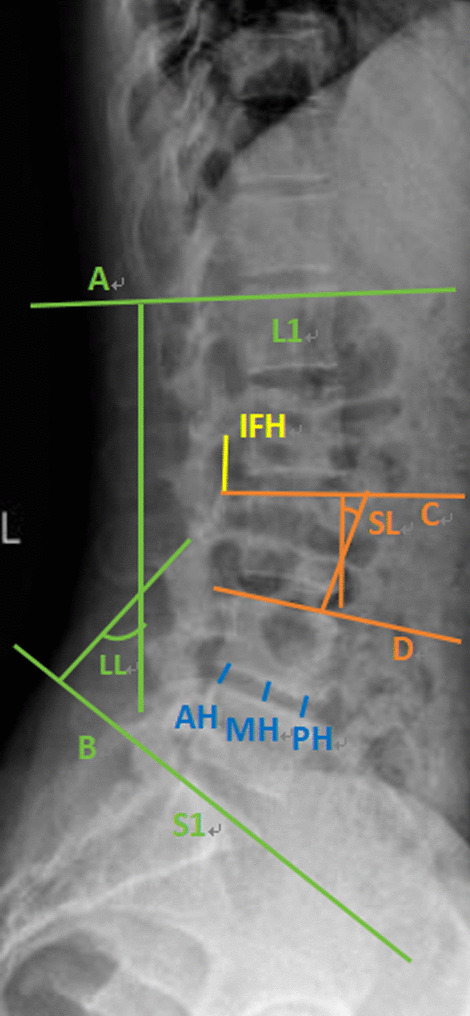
Measurement of relevant data on lateral X-ray. Lumbar lordosis angle (LL) [13] refers to the angle perpendicular to two straight lines A and B, A is parallel to the endplate of L1 vertebra, and B is parallel to the endplate of S1 vertebra. The segmental lordosis angle (SL) refers to the angle perpendicular to the two straight lines C and D, C is the parallel line to the upper endplate of the vertebral body, and D is the parallel line of the superior endplate of the inferior vertebral body. The intervertebral foramen height (IFH) is the vertical line between the lower edge of the pedicle of the upper vertebral body and the upper edge of the pedicle of the lower vertebral body, that is, a yellow straight line. The height of sagittal intervertebral space (S-ISH) = (AH + MH + PH)/3. RH, MH and LH are the heights of anterior, middle and posterior intervertebral space, respectively
